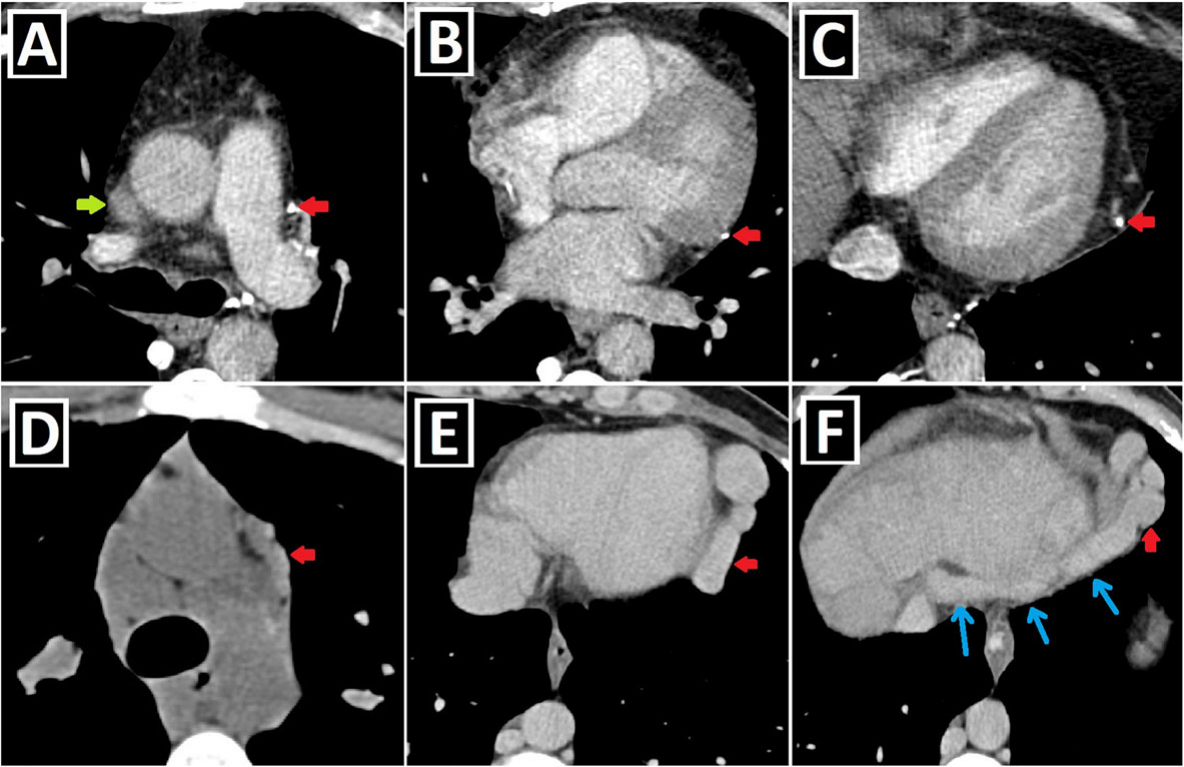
Left pericardiophrenic vein. a–c Axial CT images show a vascular structure compatible with PCPV (red arrows) in the left half of the mediastinum in a patient with SVC stenosis (green arrow). d–f Axial non-enhanced (d) and enhanced (e, f) CT images of a patient with portal hypertension secondary to Budd-Chiari syndrome depict varicose veins compatible with PCPV (red arrows) in the left half of the mediastinum. These vascular structures are connected with hepatic veins via a transdiaphragmatic course (blue arrows) (f)
